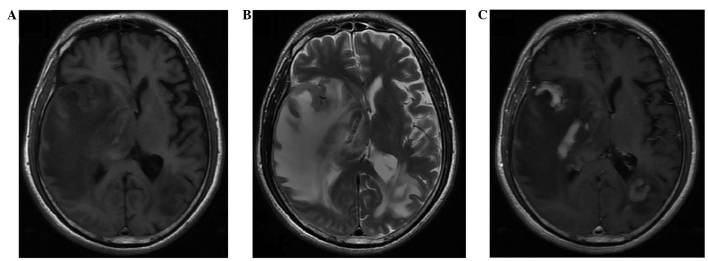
Brain MRI: Axial MRI revealed multiple expansive lesions in the right temporoparietal lobe and left parietal-occipital lobe, which showed (A) hypointensity on axial T1-weighted images, (B) hyperintensity on axial T2-weighted images and (C) homogeneous enhancement on T1-weighted contrast-enhanced MRI. The majority of the lesions showed C-shape enhancement on T1-weighed contrast-enhanced MRI. Extensive perifocal edema was also observed. MRI, magnetic resonance imaging.
